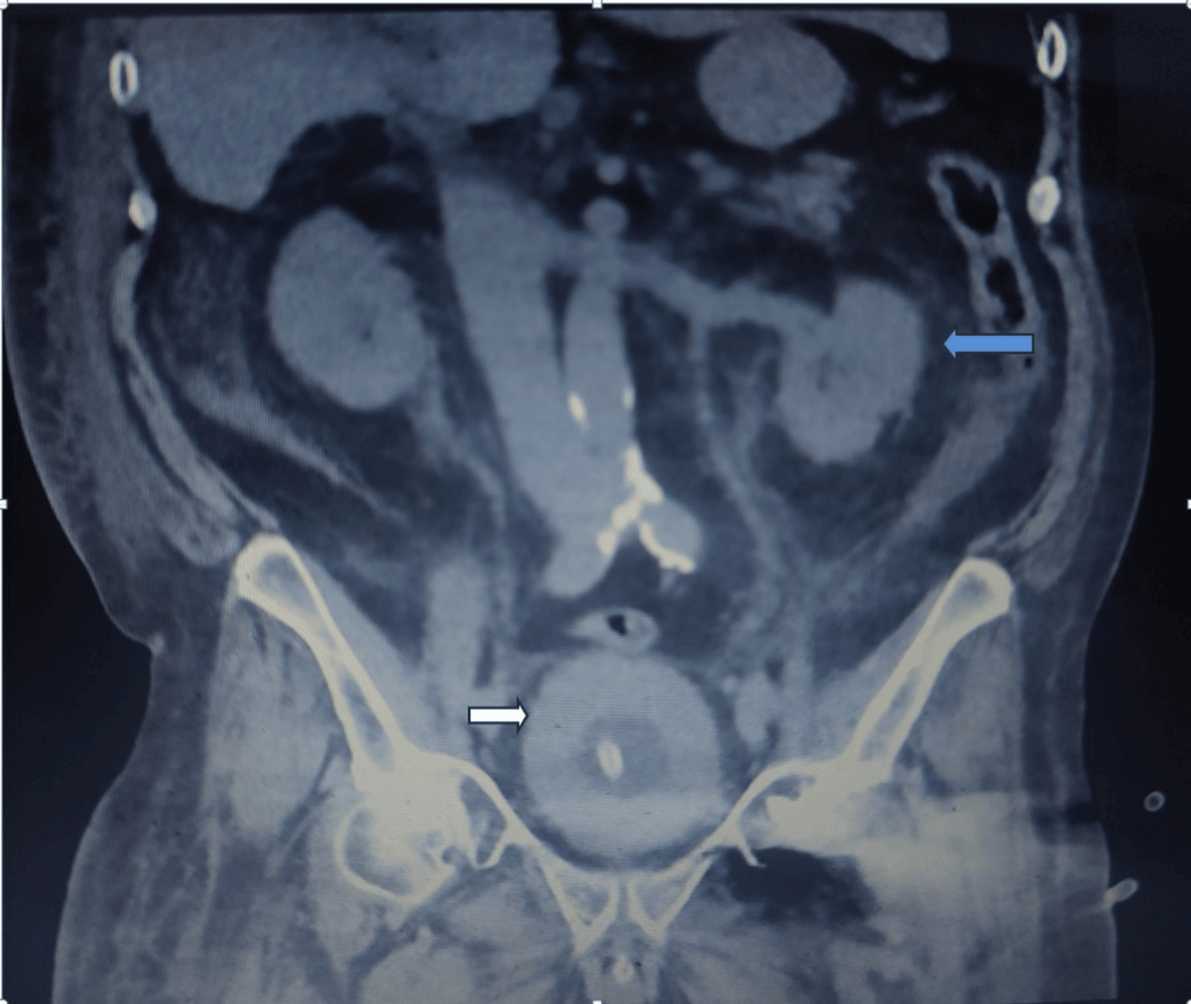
CT of the kidney, ureters and bladder showing marked bladder wall thickening (white arrow) and left hydronephrosis (blue arrow)
